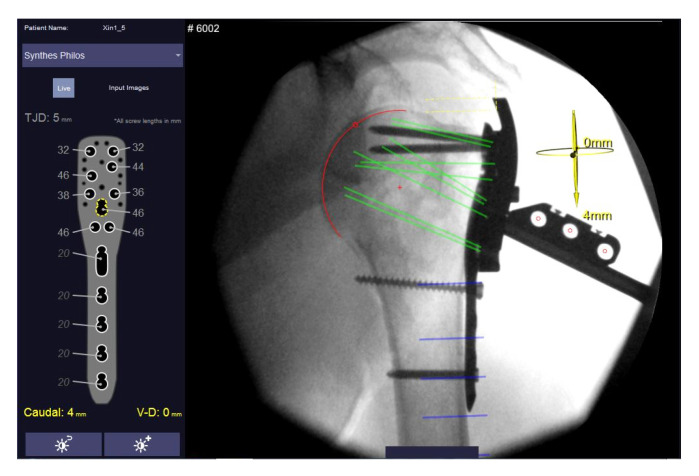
Intraoperative X-ray of one case with tilted screws (PHILOS A-level) as apparent by non-alignment with the calculated screw trajectories (green lines).
